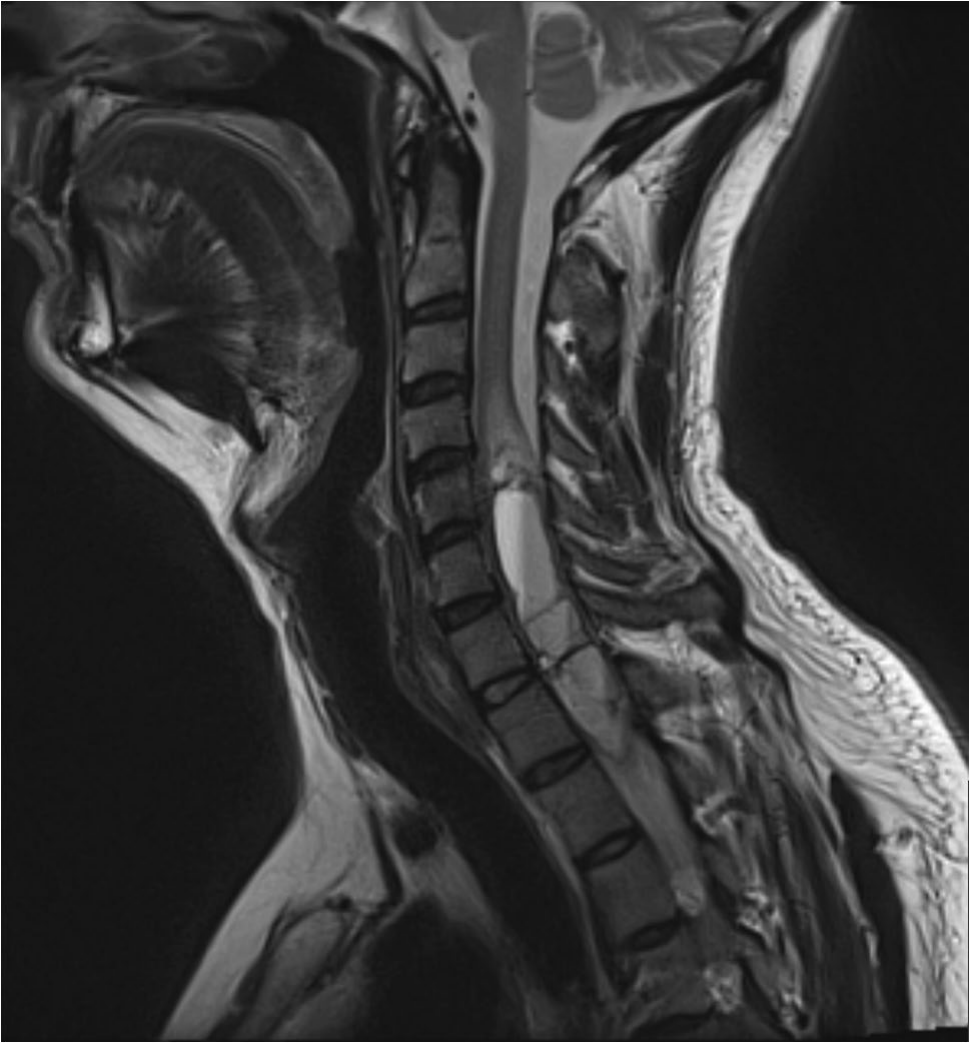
Pre-operative T2-weighted sagittal MRI of the cervical spine showing a hyperintense cystic intradural intramedullary lesion spanning from C4 to T2 and expanding the cord
